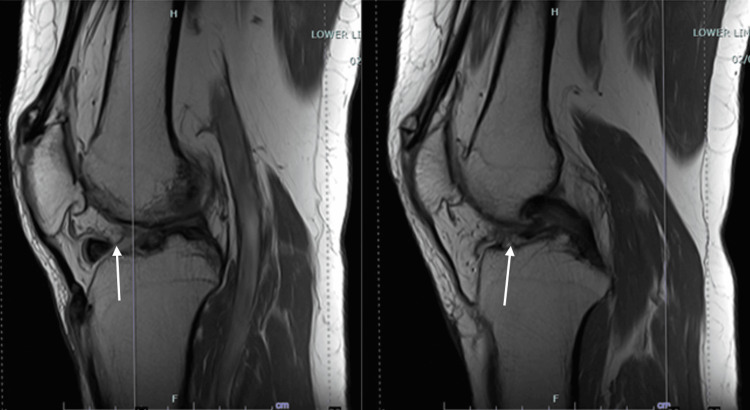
Sagittal view of the MRI, demonstrating the location of the loose body and tibial osteophyte
